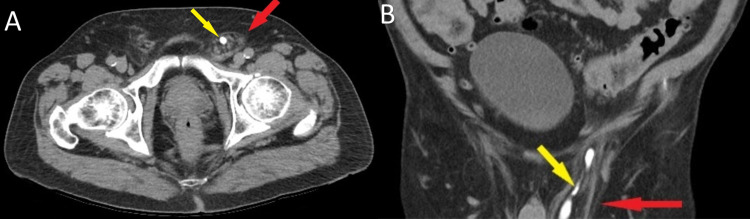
Preoperative CT scan of the abdomen and pelvis (A, B) Left inguinal hernia (red arrow) containing ureter (yellow arrow)
